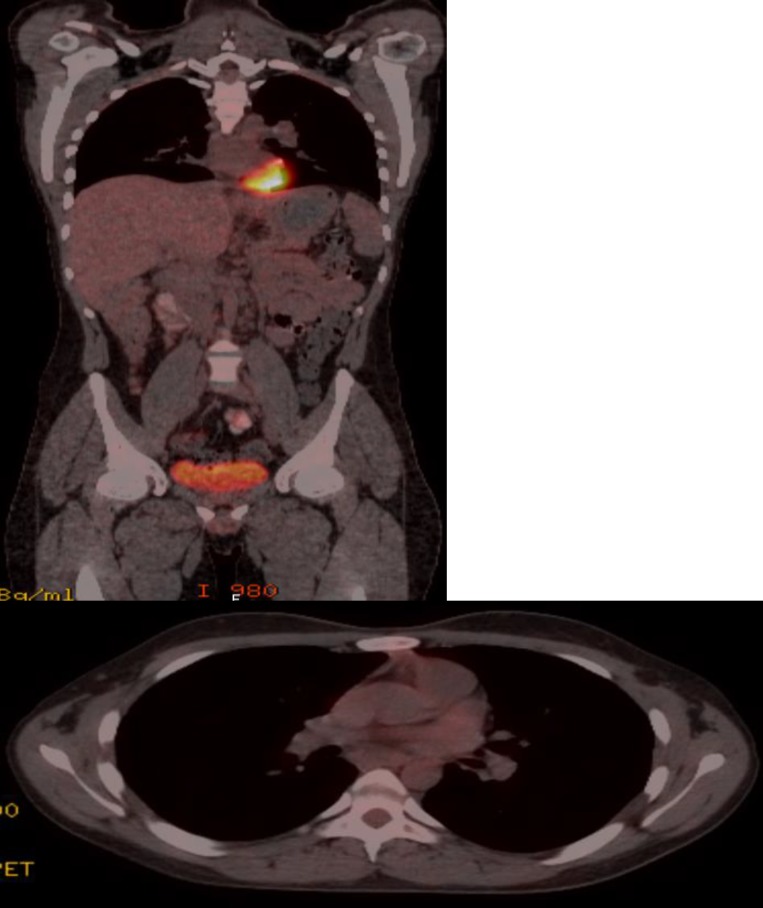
PET/CT showed bilateral a few 1 to 2 cm diameter cervical lymphadenopathies without FDG uptake. It was interpreted as complete metabolic remission.
